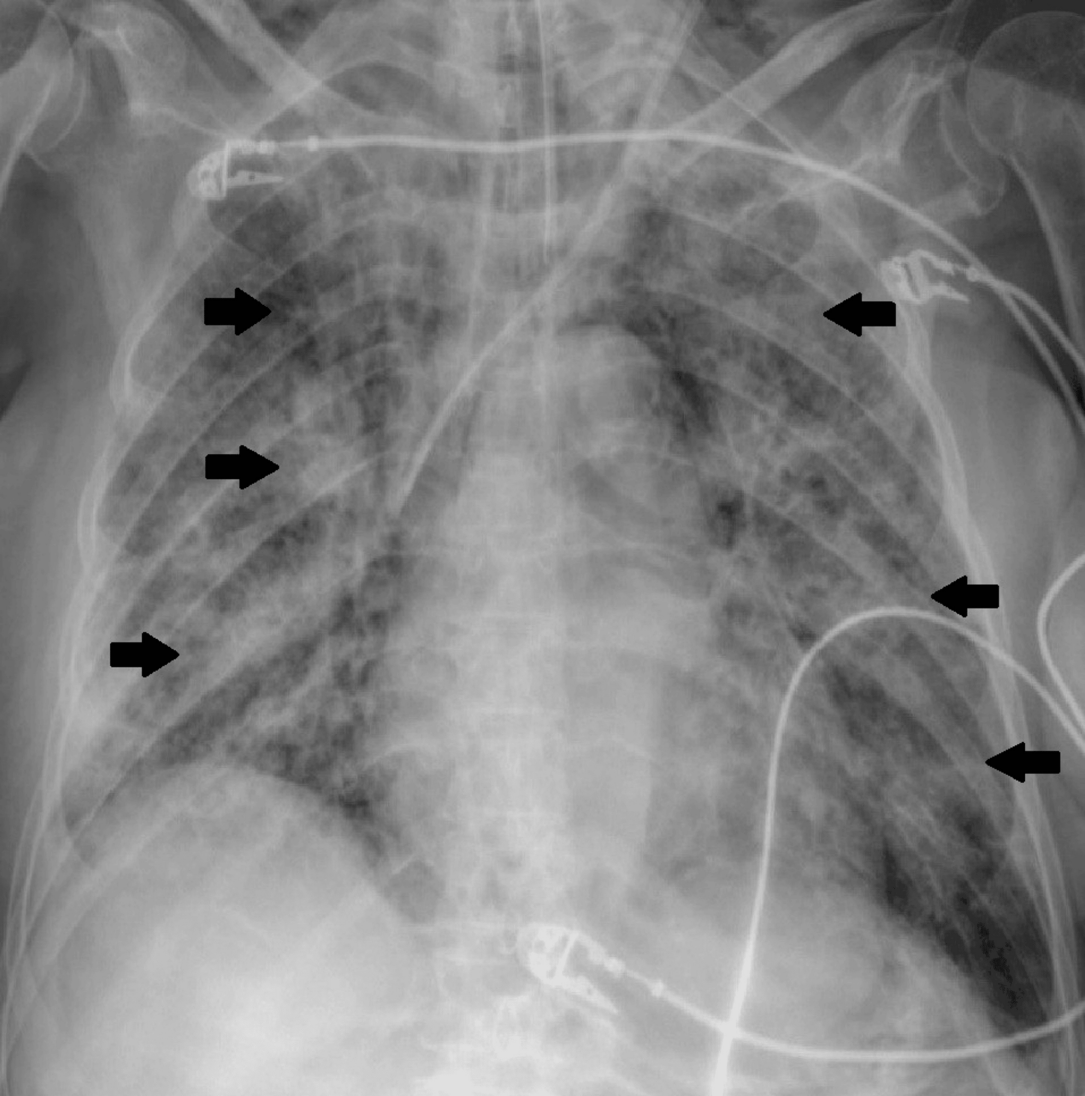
Chest X-ray after worsening of clinical condition. The image shows pneumonia COVID-19 and diffuse lung opacities.
